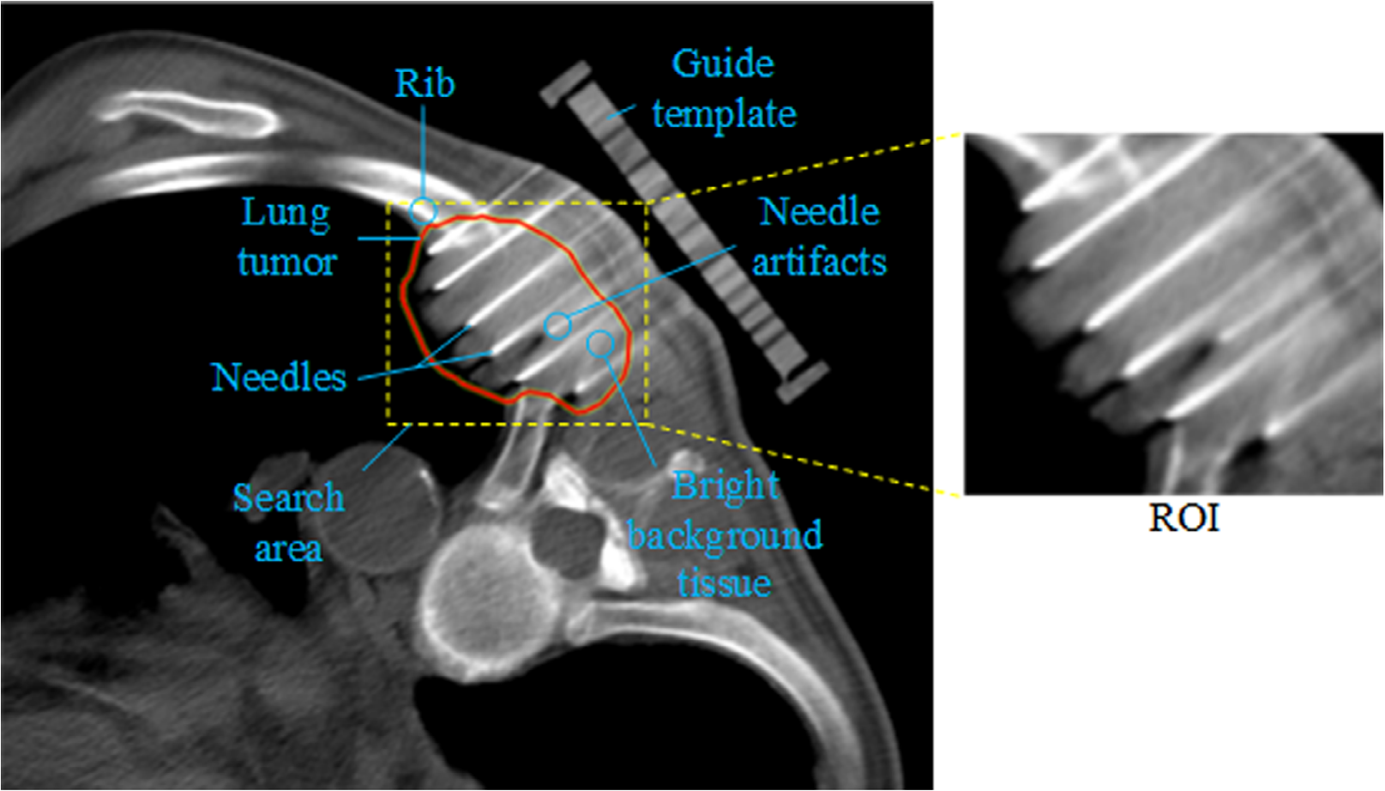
An instance of the needle appearance during lung interstitial brachytherapy performed on CT images. The ROI is displayed on the right.
